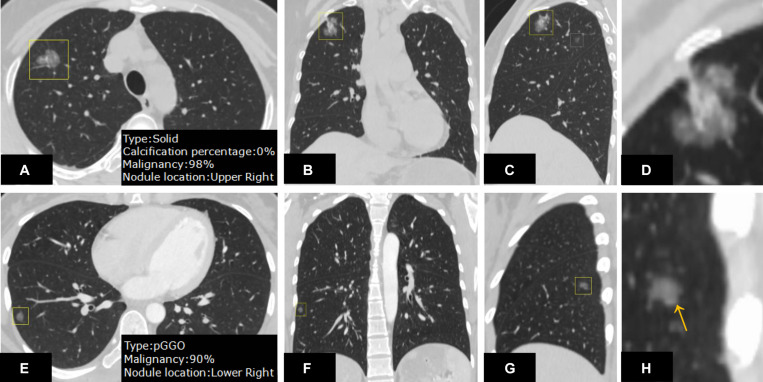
On the CT images from different views (A–C) and zoom-in view (D), a nodule in the right upper lobe was detected. DL-CADx subtyped this nodule as a solid nodule, while double reading characterized it as a part-solid nodule. By integrating these two disagreed results, a final consensus that this is a part-solid nodule has been reached through discussion. Differently, for the nodule detected on the CT images from different views (E–G) and zoom-in view (H), the first impression by double reading was a part-solid nodule in the left upper lobe because of seemingly a small patch of solid component (arrow). However, DL-CADx classified it as ground-glass nodule. After double checking and discussion, we misinterpreted a small vessel (arrow) inside the nodule as solid component and reached an agreement that this is a ground-glass nodule.
